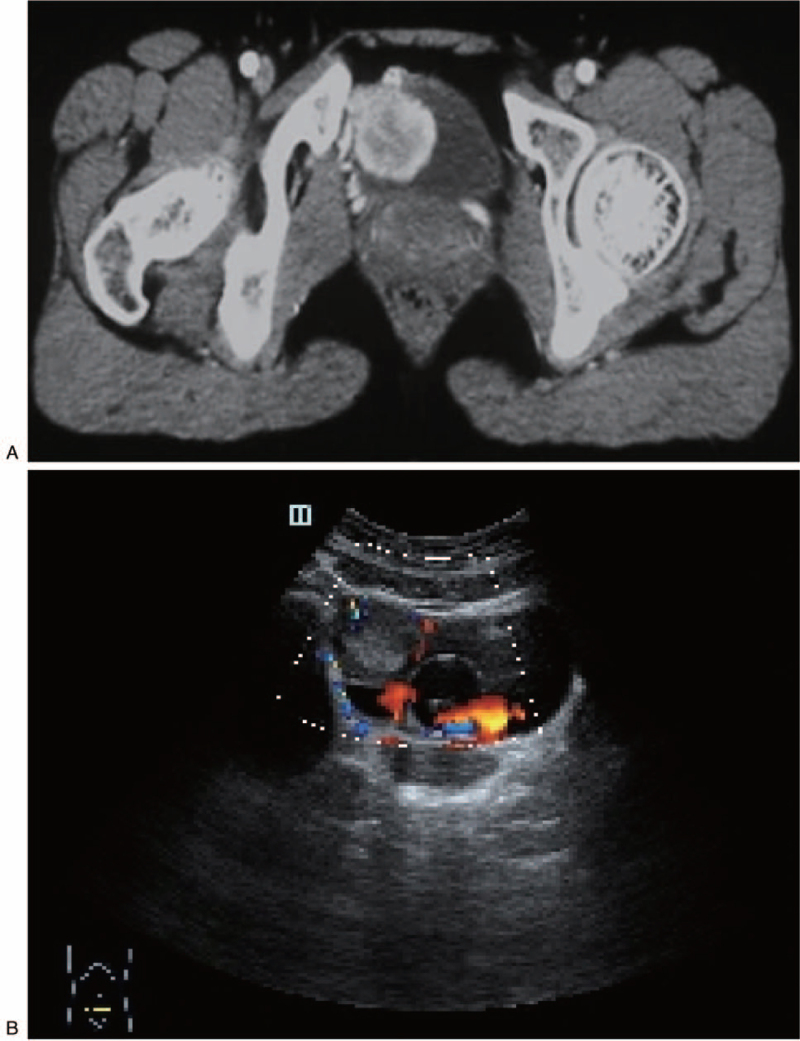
Images show a tumor at the right side of the anterior bladder wall. (A) Contrast-enhanced computed tomography revealed a mass in the bladder. (B) Ultrasound imaging revealed a mass in the bladder.
